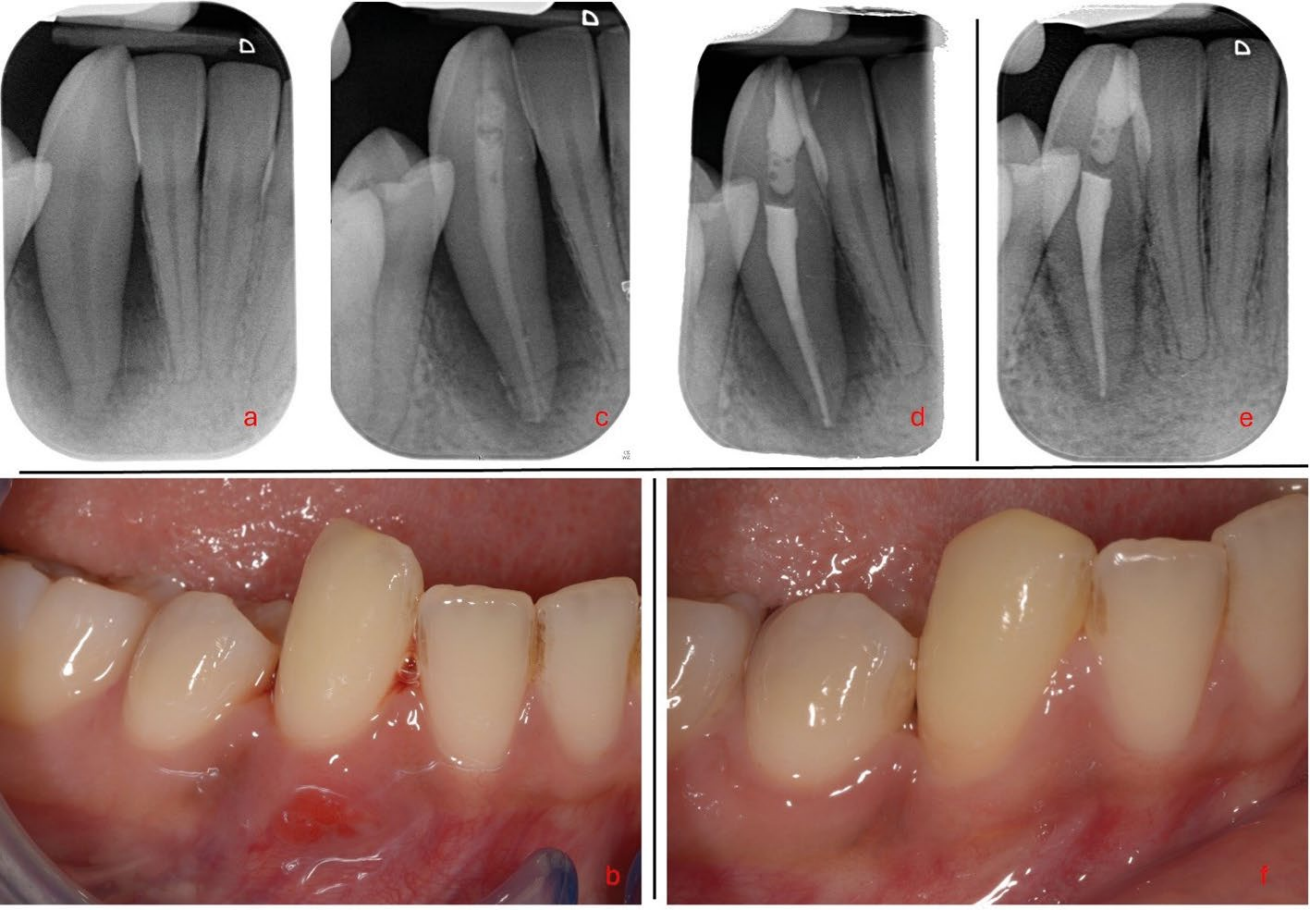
Case of localised periodontitis in a young patient, including an EPL affecting tooth 43. Initial presentation of tooth 43 with deep periodontal probing depths >6 mm affecting buccal lingual and distal aspects of the tooth combined with diffuse apical pathology combined with marginal bone loss. a, b) The tooth was also over-erupted but not mobile. c, d) RCT was undertaken over two visits, with recontouring of the crown to improve appearance. Targeted subgingival PMPR was undertaken immediately after completion of the RCT. e, f) At six-month review, probing depths had reduced to 4 mm with resolution of the inflammation, no bleeding on probing and radiographic bone fill seen. The tooth was placed into a maintenance phase
